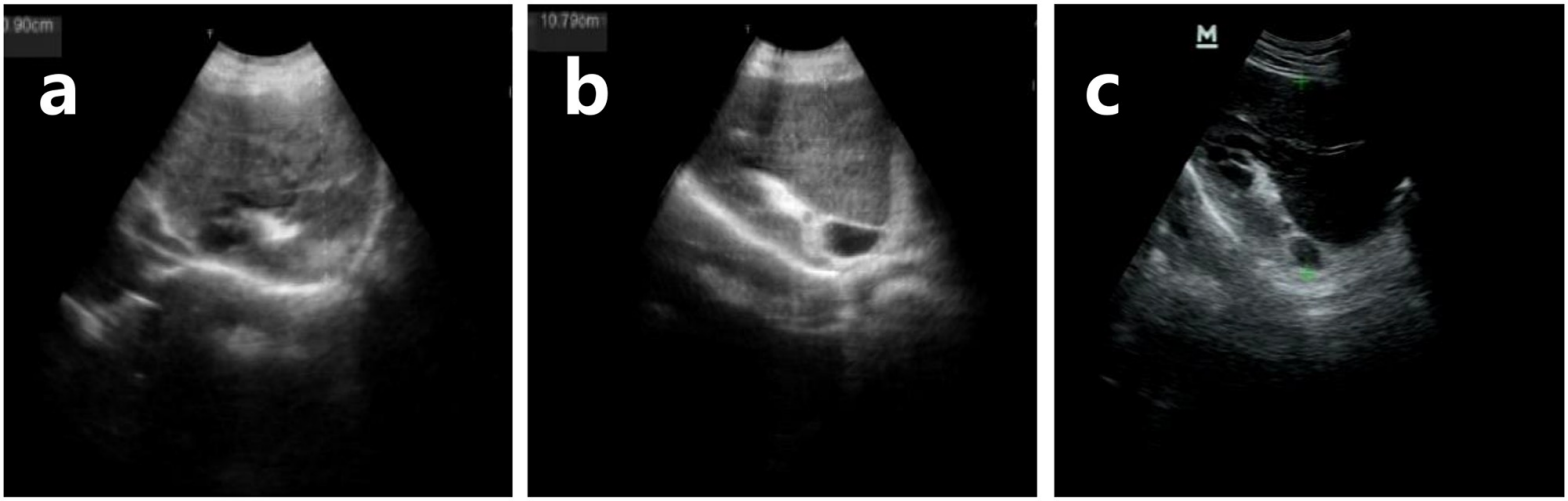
Reversal of liver fibrosis from Grade 3 to 1 within 3 years in a female patient with advanced schistosomiasis after splenectomy in Nanling (Anhui, China). (a) Grade 3, severe fibrosis (checked on 14 May 2015). (b) Grade 2, moderate fibrosis (6 July 2016). (c) Grade 1, mild fibrosis (9 January 2018). The ultrasound examination was performed by the same technician using Teknova for image a and b and Mindray for image c in the Schistosomiasis-specialized Hospital of Nanling (Anhui, China).
